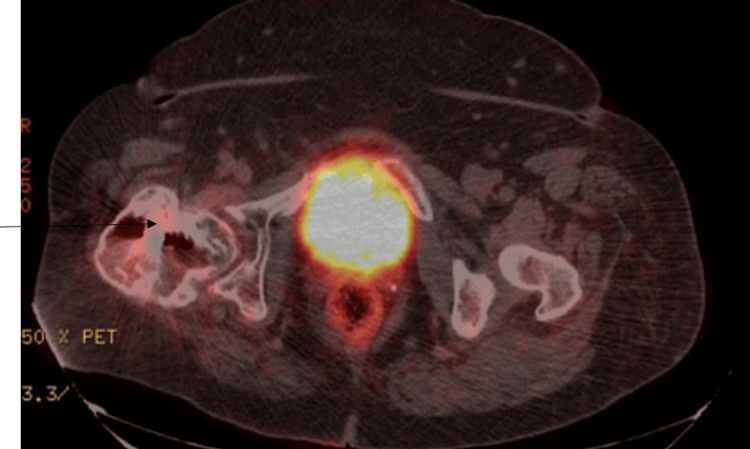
PET/CT showing right-sided femoral expansible lesions. The arrow points to the increased uptake in the right-sided femoral region on the PET scan. PET/CT = positron emission tomography/computed tomography
